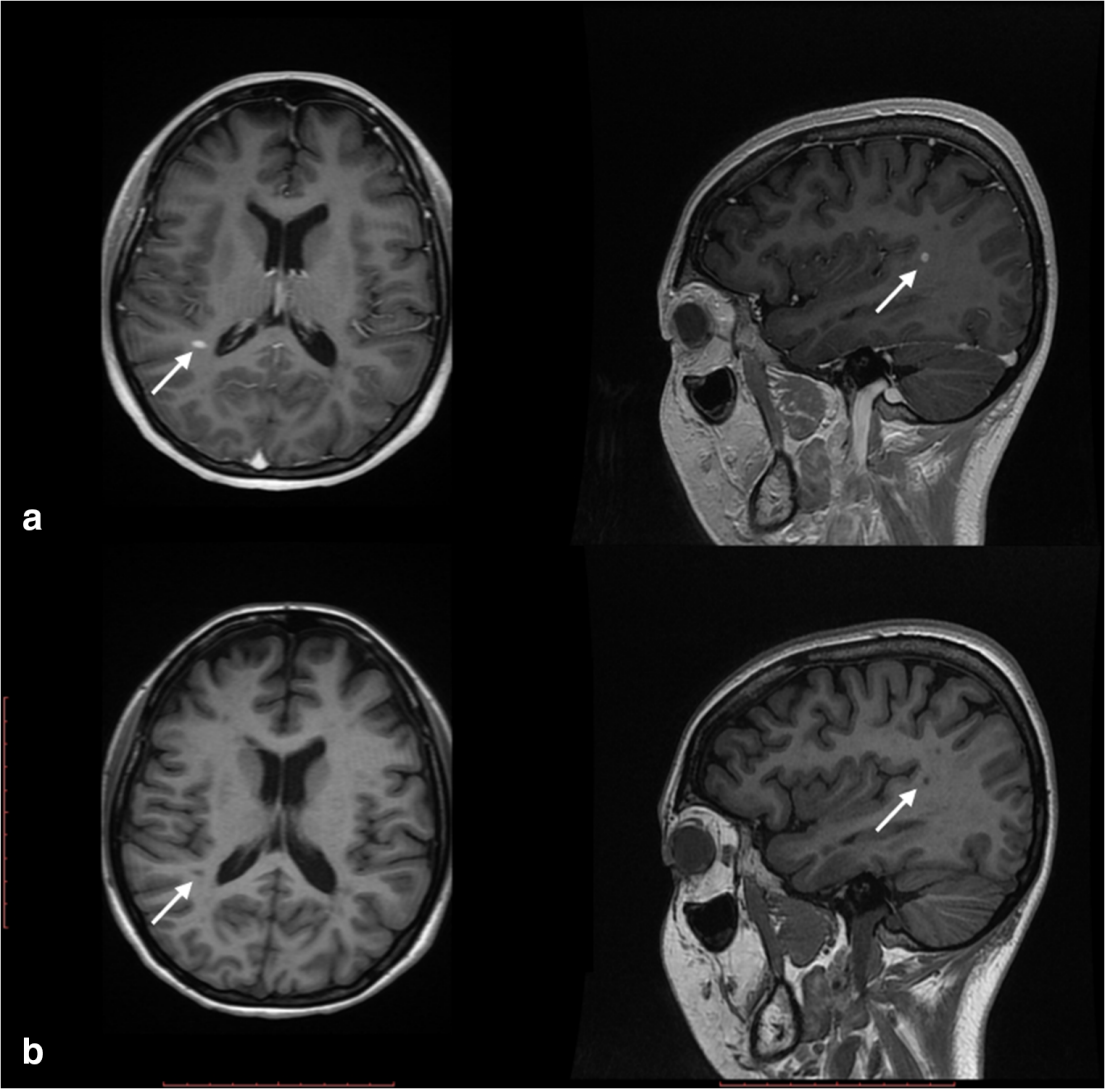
a Contrasted brain MRI T1 sequences (2017). High uptake images along the supramarginal gyrus in the caudal aspect of the left parietal lobe (white arrows). b No contrasted brain MRI T1 sequences (2017). Hypointense sphere-like image in the caudal aspect of the left parietal lobe (white arrows)
